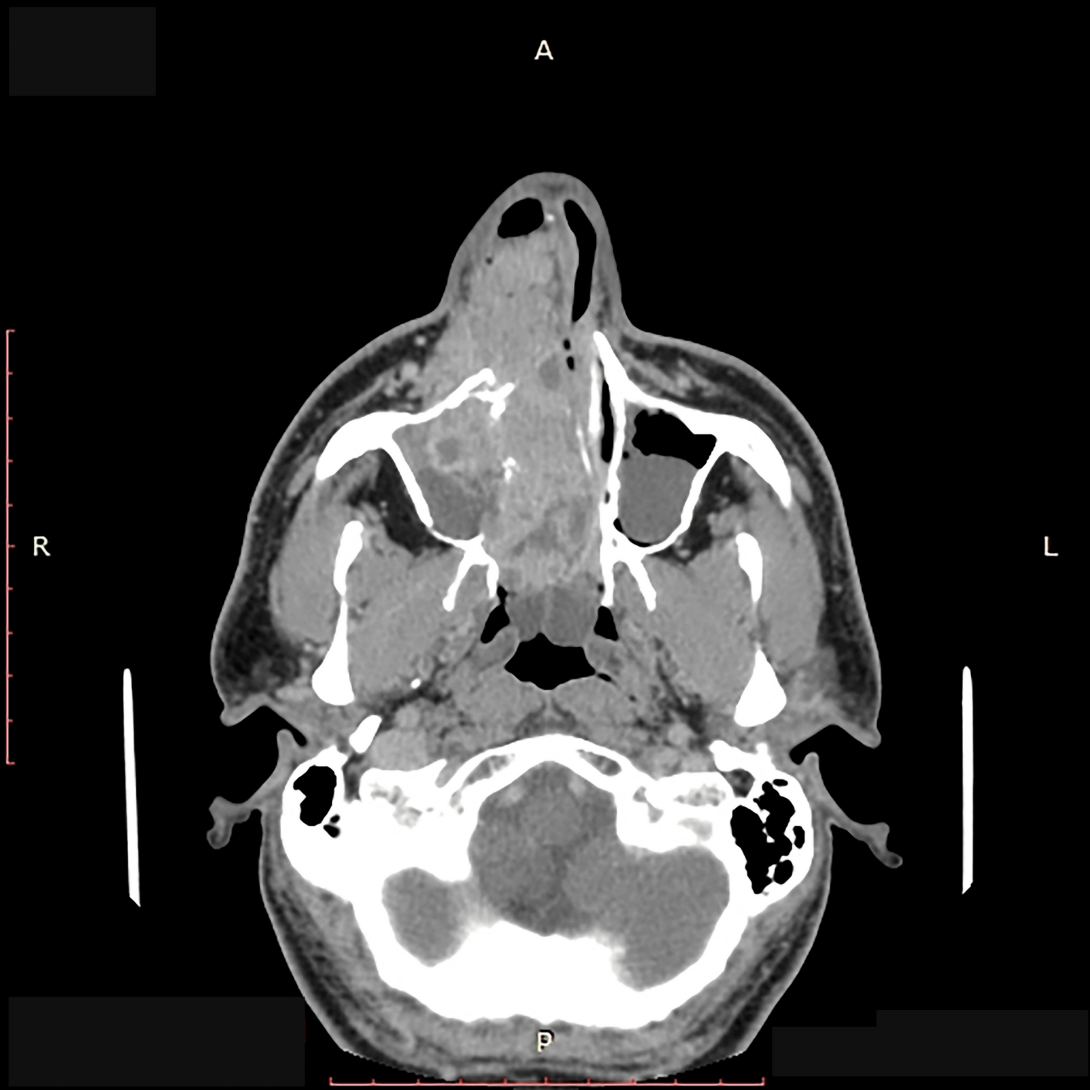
Computer tomography (CT) scan of the paranasal cavities L-left; R-right Tumor infiltration with bone destruction of the medial wall of the maxillary sinus, the frontal process of the maxillary bone, and the medial wall of the orbit, on the right side. The nasal septum was severely compressed and displaced by the tumor mass contralaterally.
